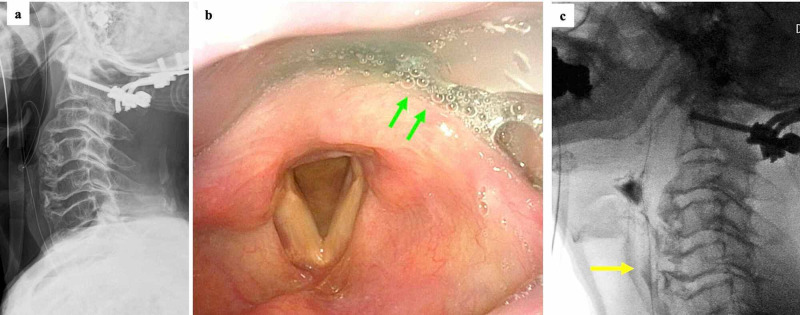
Postoperative images Lateral radiograph (a) after occipito-cervical posterior fusion. Swallowing videoendoscopy (b) on the second day after surgery showing stagnation of thick fluid at the larynx (arrows). Swallowing fluorography (c) in the fourth week after surgery showing improvement of swallowing without stagnation (arrow)
